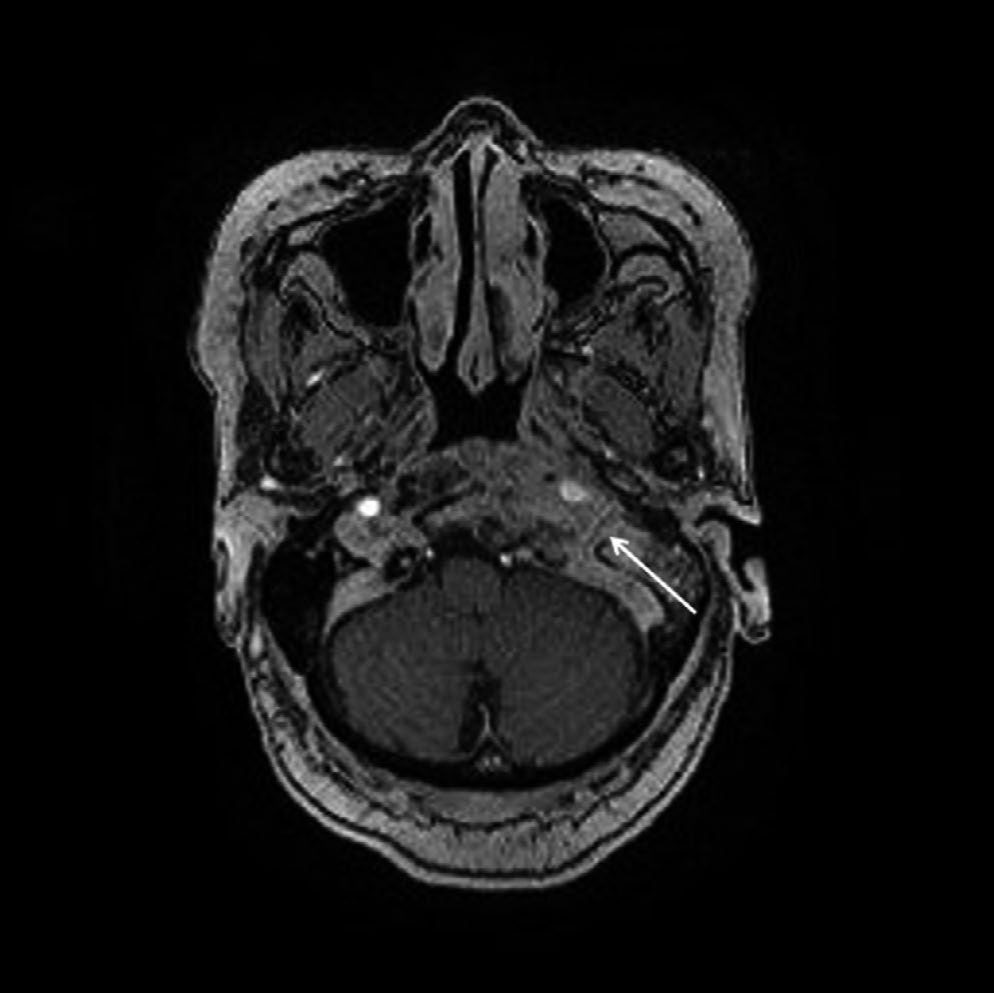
T1‐weighted MRI with contrast showing left clival, prevertebral, and petrous mass obscuring left jugular bulb (open arrow)
